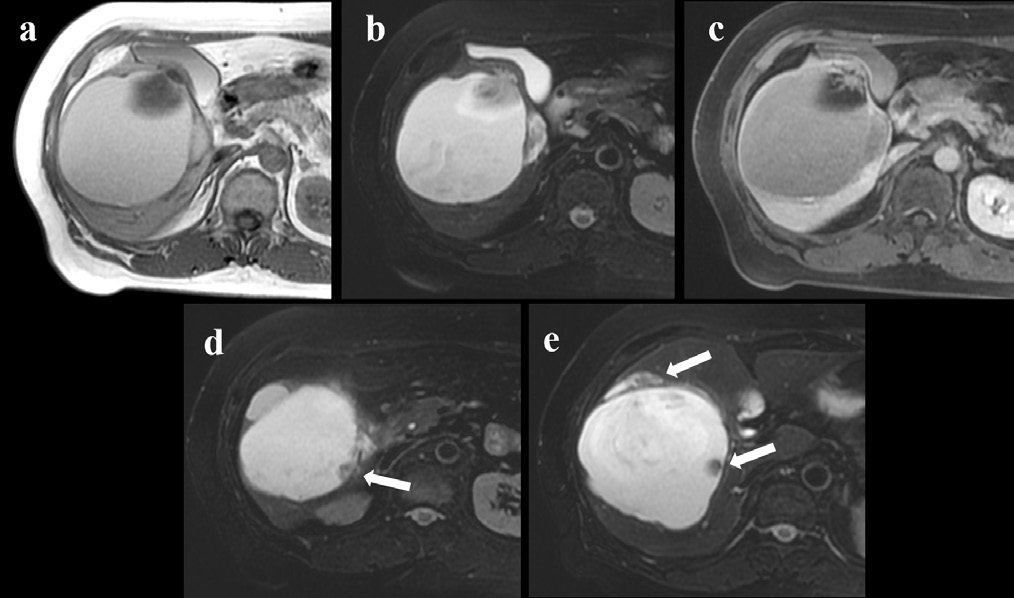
Abdominal magnetic resonance (MR) imaging. (a) Axial T1-weighted image shows the cysts of high intensities, suggestive of the presence of a mucin-containing tumour. (b) Axial T2-weighted fat suppressed image shows cysts with septation and the mural nodule. (c) Axial Gd-enhanced T1-weighted image shows an enhanced mural nodule. (d–e) Axial T2-weighted fat suppressed image shows several mural nodules in the cystic mass (arrows)
